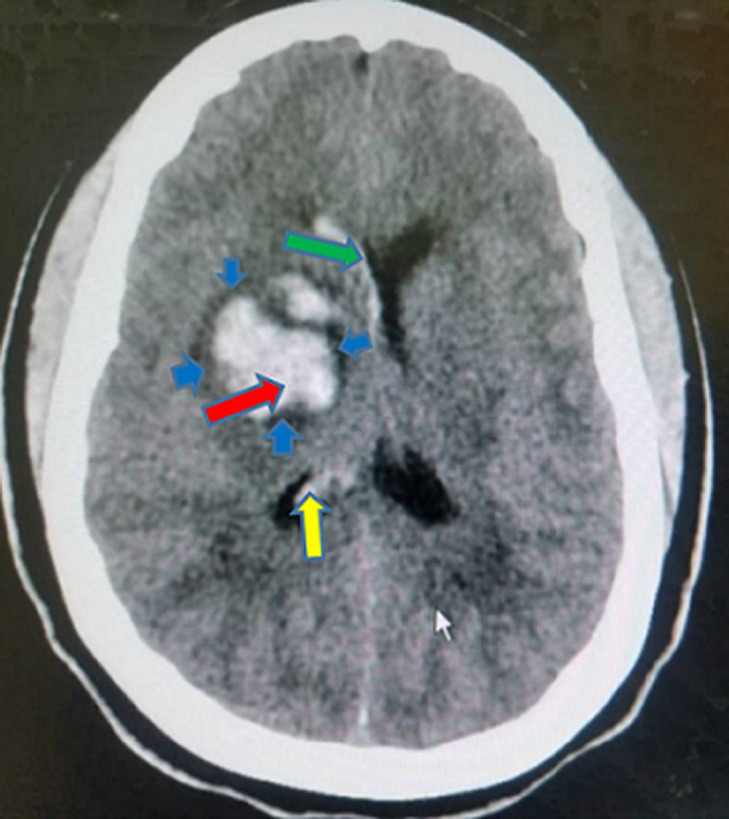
a non-contrast axial CT scan of the head showing an acute right basal ganglia hemorrhage (red arrow), perilesional edema (blue short arrows), extension into the ipsilateral lateral ventricle (yellow arrow) and mass effect (green arrow)
